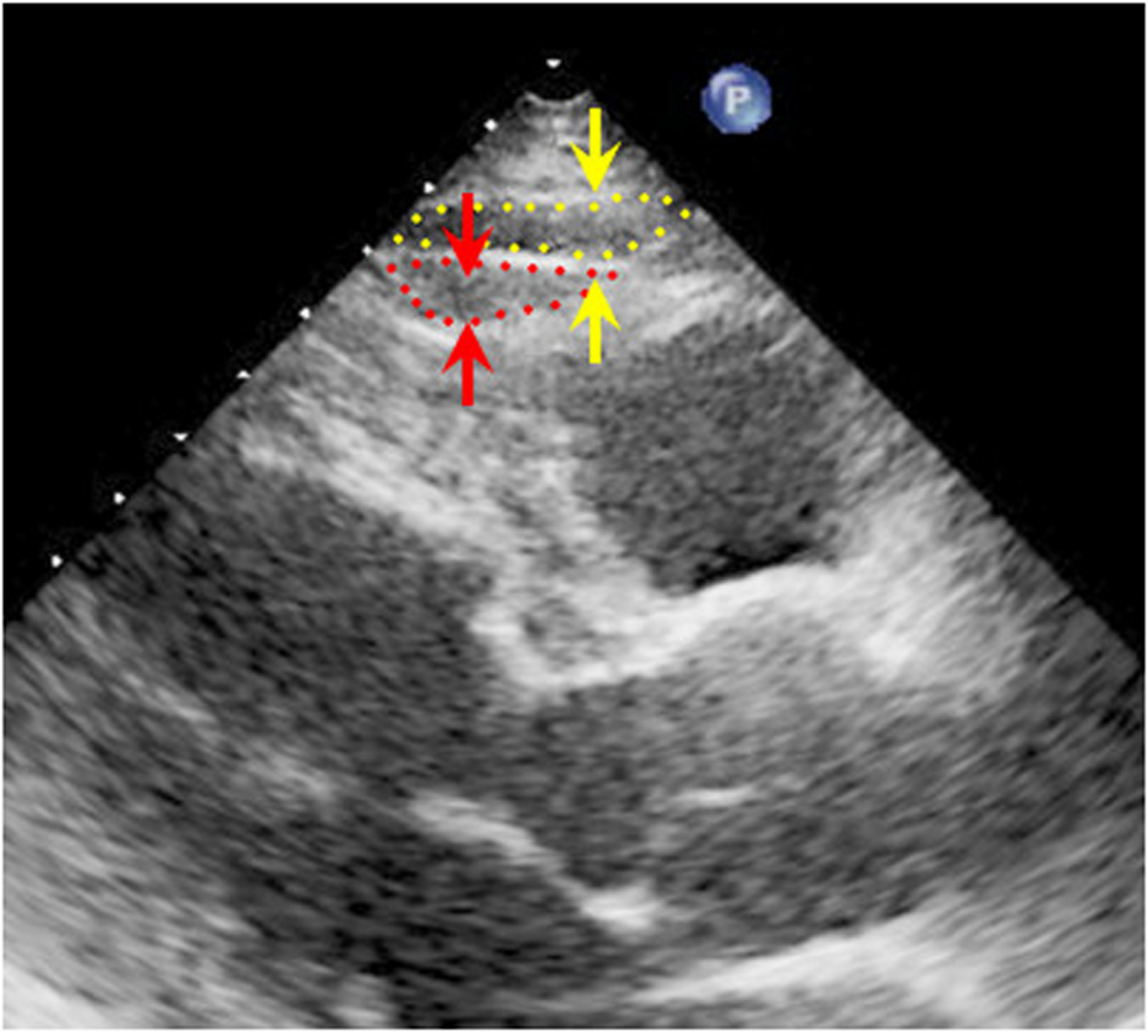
Echocardiographic images of a 55-year-old male patient with hypertension, dyslipidemia, and diabetes. The red area is the epicardial fat within the pericardial sac as quantified 3-dimensionally by echocardiography.
